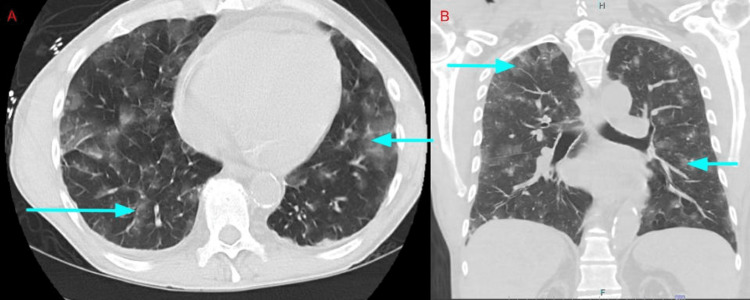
CT of the chest without contrast revealing bilateral nodular ground-glass opacities (blue arrows pointing to ground-glass opacities) compatible with alveolar hemorrhage.
